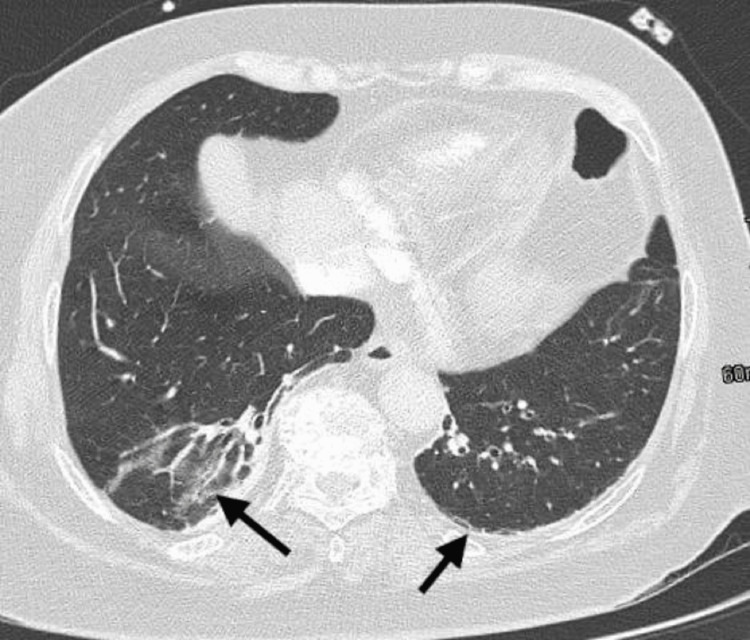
CT angiography of the chest showing bilateral atelectatic changes of the lower lobes (right >>left). The rest of the lung parenchyma is clear; no masses or consolidations, and no suspicious nodules. CT: computed tomography.
